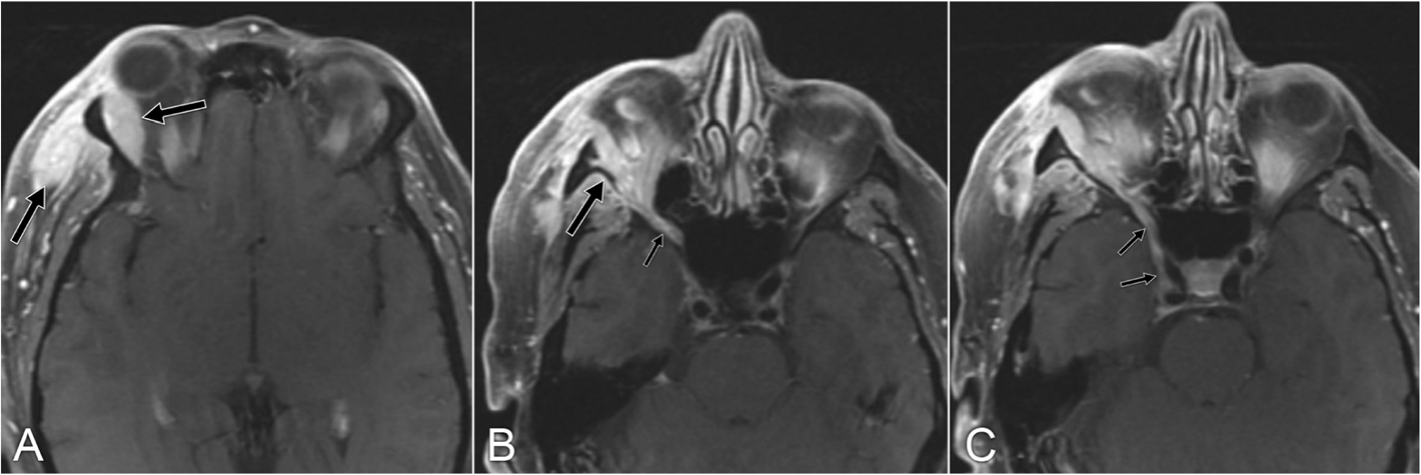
Axial postcontrast MR images of a 51-year-old-man with DLBCL who presented with diplopia (patient 5). a Mass in the right lacrimal gland and masticator space (arrows). b Extension from the orbital mass (large arrow) through the inferior orbit fissure (small arrow) into the PPF. c Involvement of the foramen rotundum and cavernous sinus (arrows)
